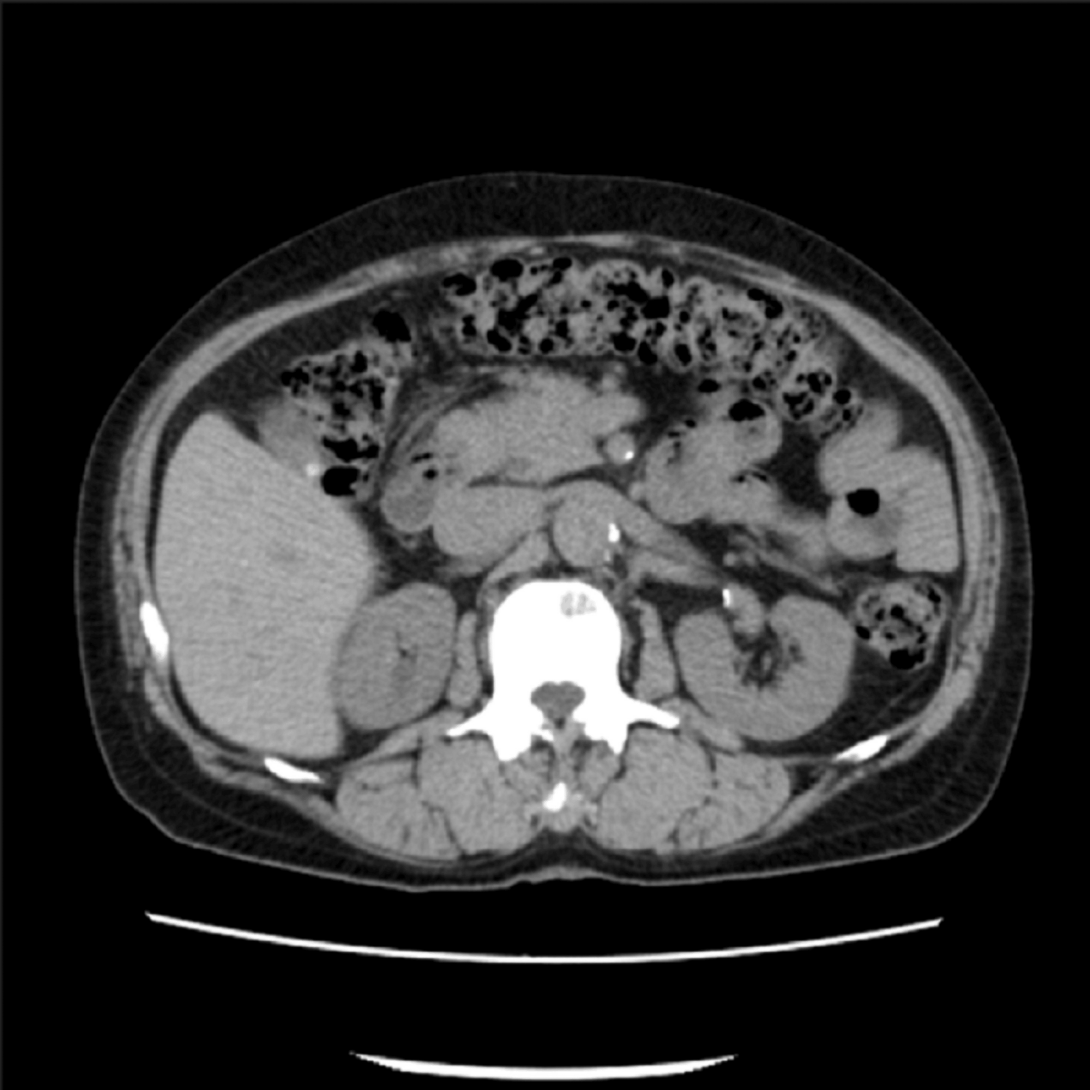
Metastatic sites in patient 1 Computed tomography imaging revealed no apparent visceral metastases.
